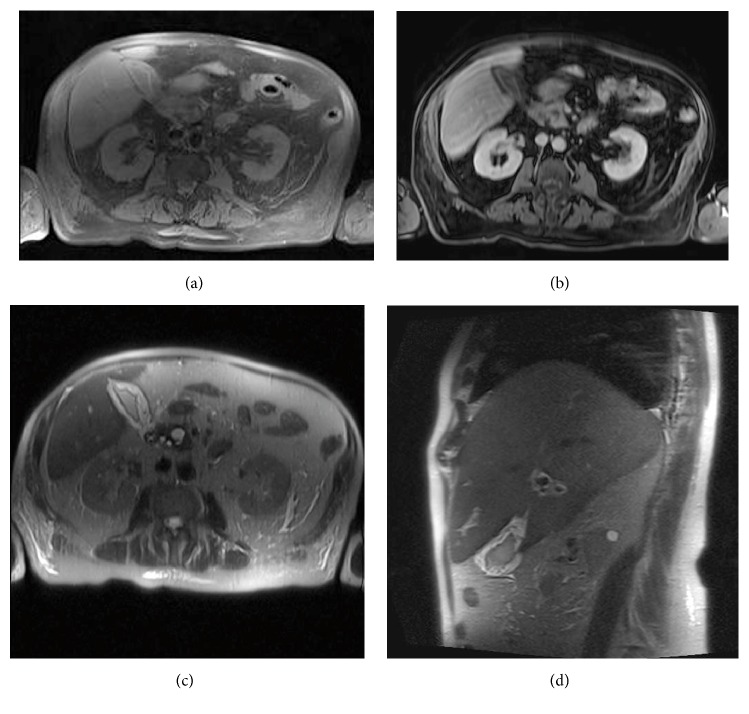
(a) Axial T1W-VIBE precontrast, (b) axial T1W-VIBE postcontrast, (c) axial T2W-fat suppressed, and (d) coronal T2W-fat suppressed sequences. MR images show diffuse gallbladder wall thickening with increased signal intensity on T2 weighted fat suppressed images. Postcontrast fat suppressed images demonstrate homogeneous mucosal enhancement with no transmural wall enhancement.
